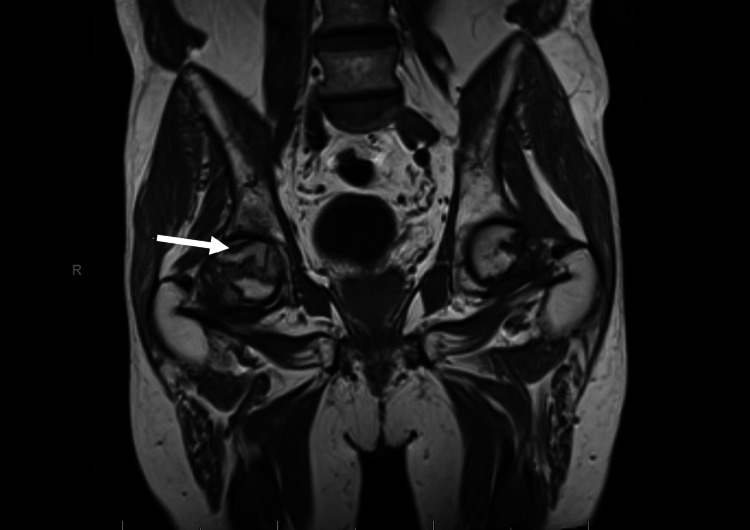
MRI pelvis showing marked marrow edema and enhancement in the right femoral neck
